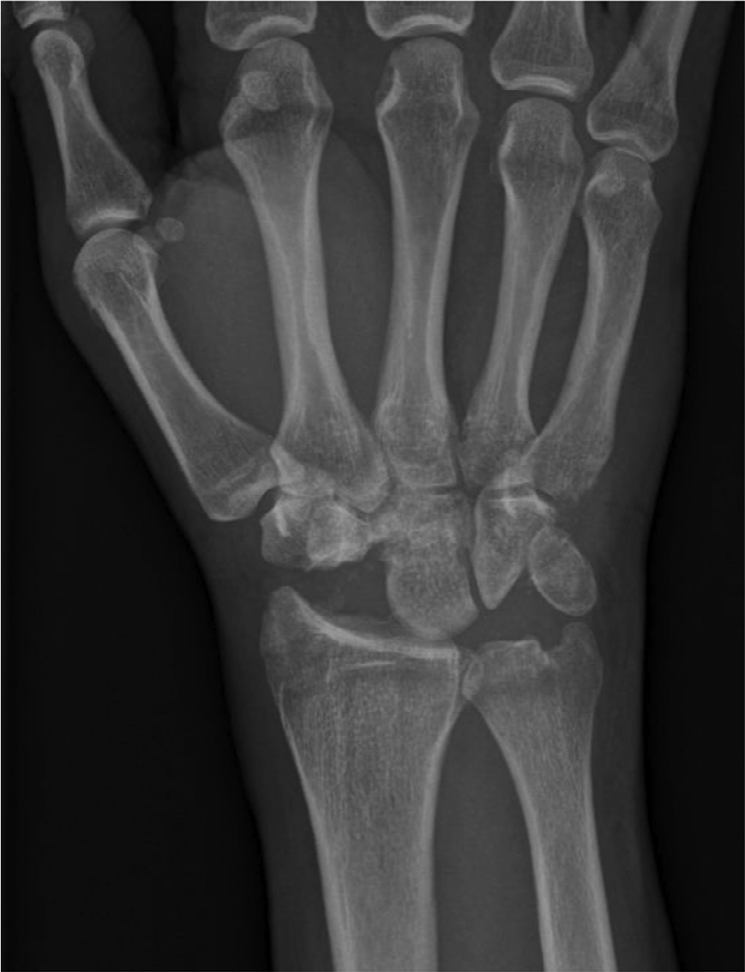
Patient 1: Postoperative radiograph of the right wrist showing changes of proximal row carpectomy.
